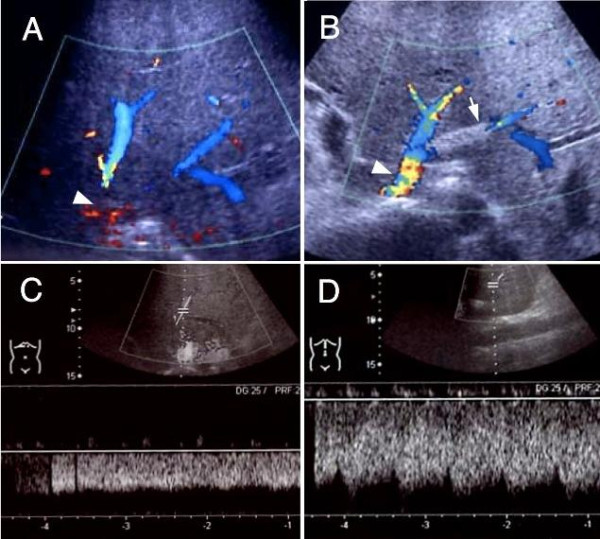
The Doppler ultrasound images before (A, C) and after (B, D) the stent placement therapy.
